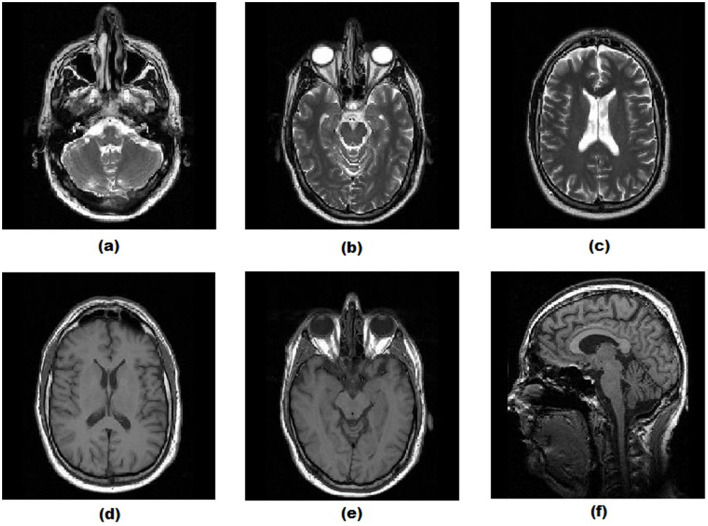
Original brain magnetic resonance images: (A) MRI Brain Image 1, (B) MRI Brain Image 2, (C) MRI Brain Image 3, (D) MRI Brain Image 4, (E) MRI Brain Image 5, and (F) MRI Brain Image 6.
